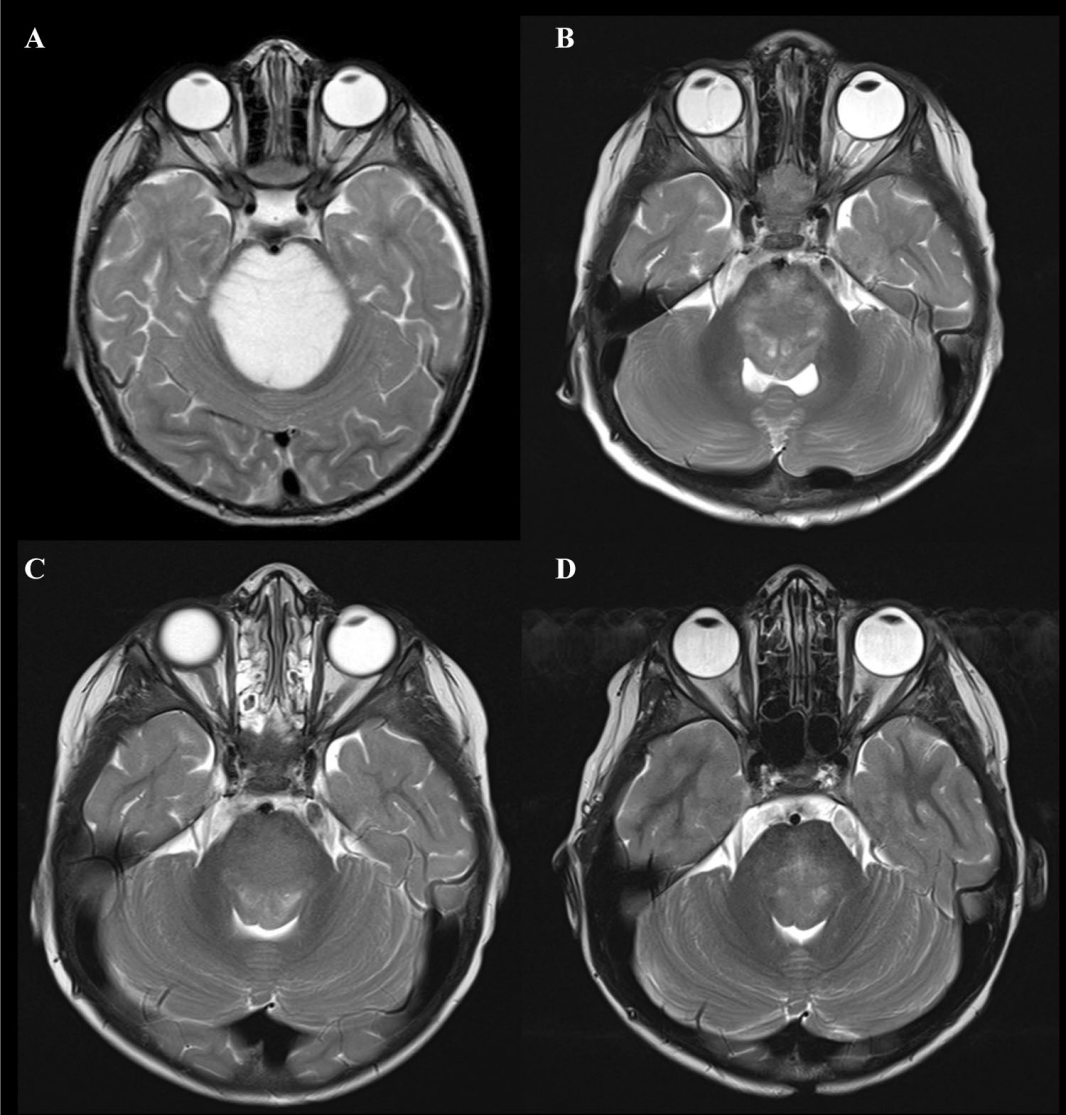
MRI demonstrating response to the therapy using T2-weighted axial images. A Tumor extent at the time of diagnosis. B Partial regression of the tumor during intensive chemotherapy (after 6 months). C At the end of intensive treatment and D continuous remission after 6.5 years from diagnosis
